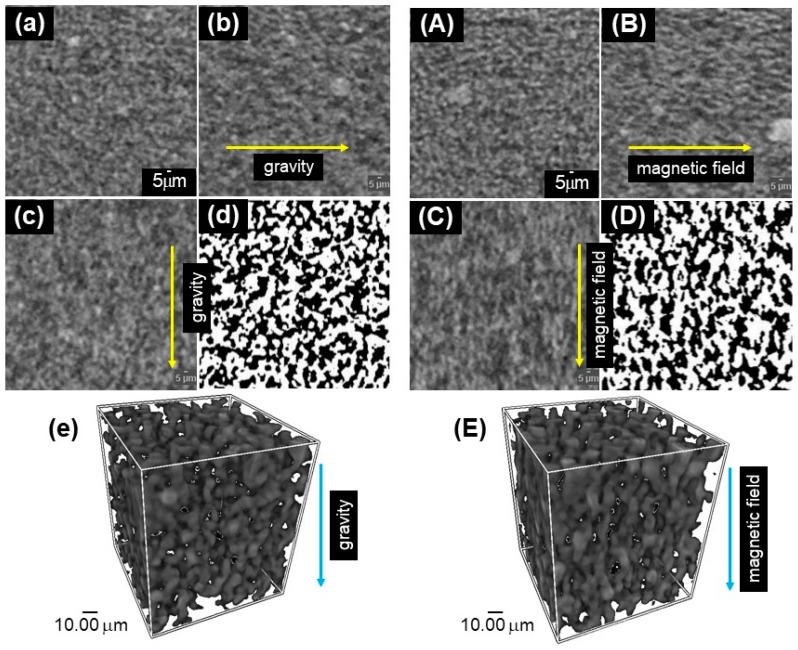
CT images for magnetic elastomers at (a–e) 0 mT and (A–E) 270 mT with different views; (a,A) top, (b,B) side, (c,C) front, and (e, E) 3D view. The directions of gravity and magnetic field are indicated by arrows. (d,D) Black-and-white images of the side view (CI 70 wt%, DOP 70 wt%).
